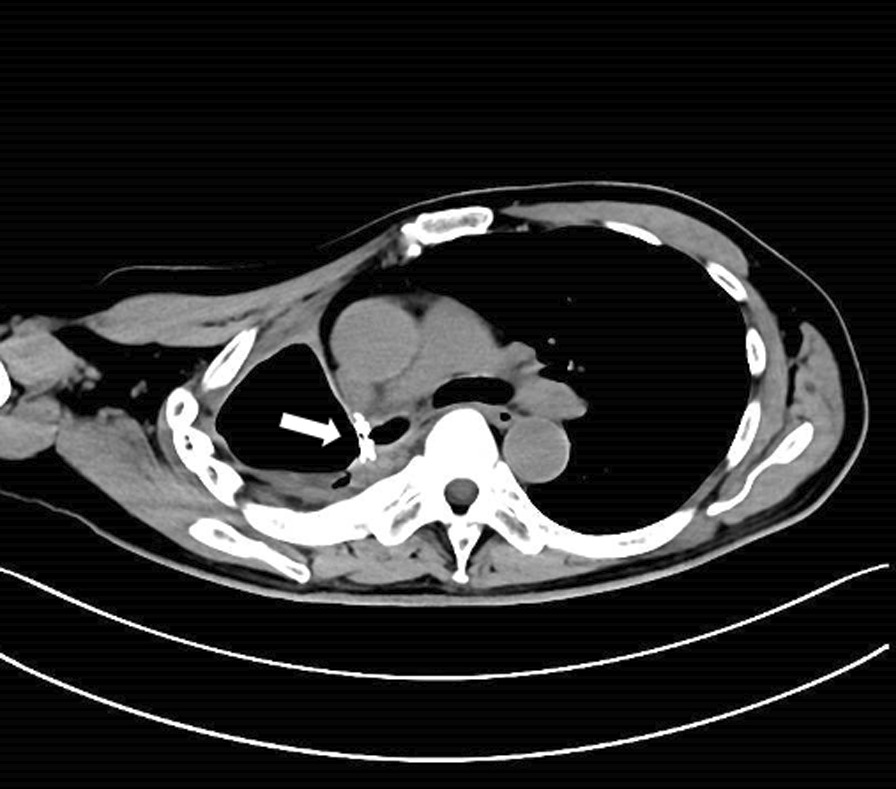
Patient no. 4 in Table 1. The BPF in the right main bronchus was completely closed by the VSD occluder (white arrow) in the latest chest computed tomography
